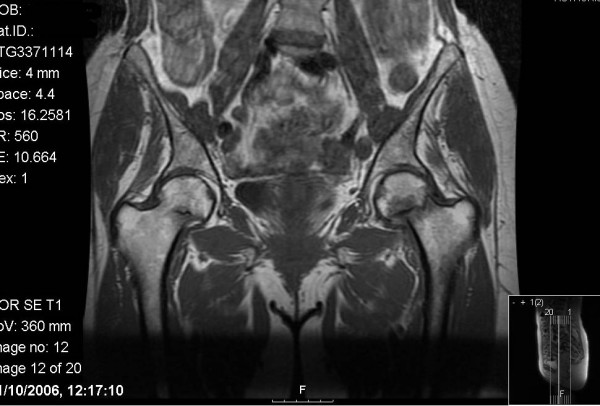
T1 weighted MRI showing bilateral undisplaced neck of femur fractures. This investigation was performed six weeks after the onset of symptoms.
